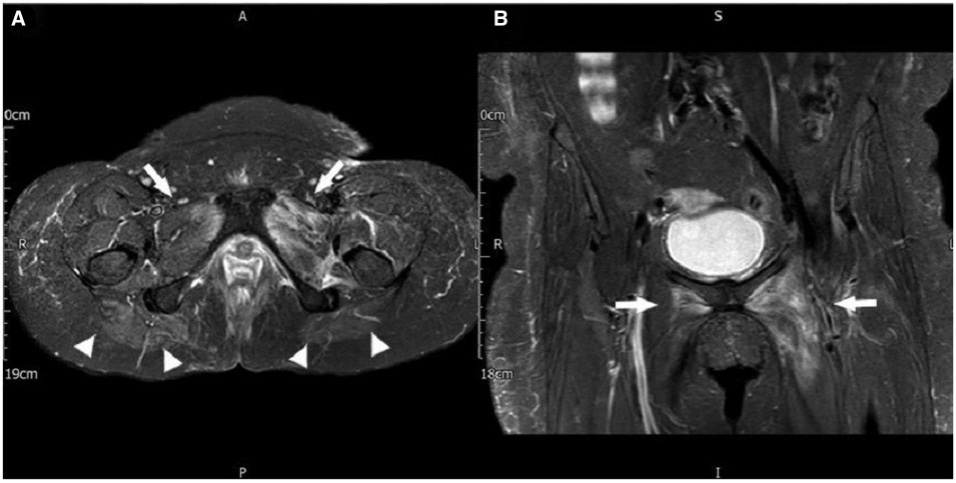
MRI showing multifocal patchy muscle oedema Short tau inversion recovery transverse (STIR _TRA; A) and Short-TI Inversion Recovery Coronal (STIR _COR; B), Showing multifocal areas of patchy oedema centred within the adductor compartment bilaterally (arrows) and the medial, superior aspect of the gluteal muscles bilaterally (arrowheads).
